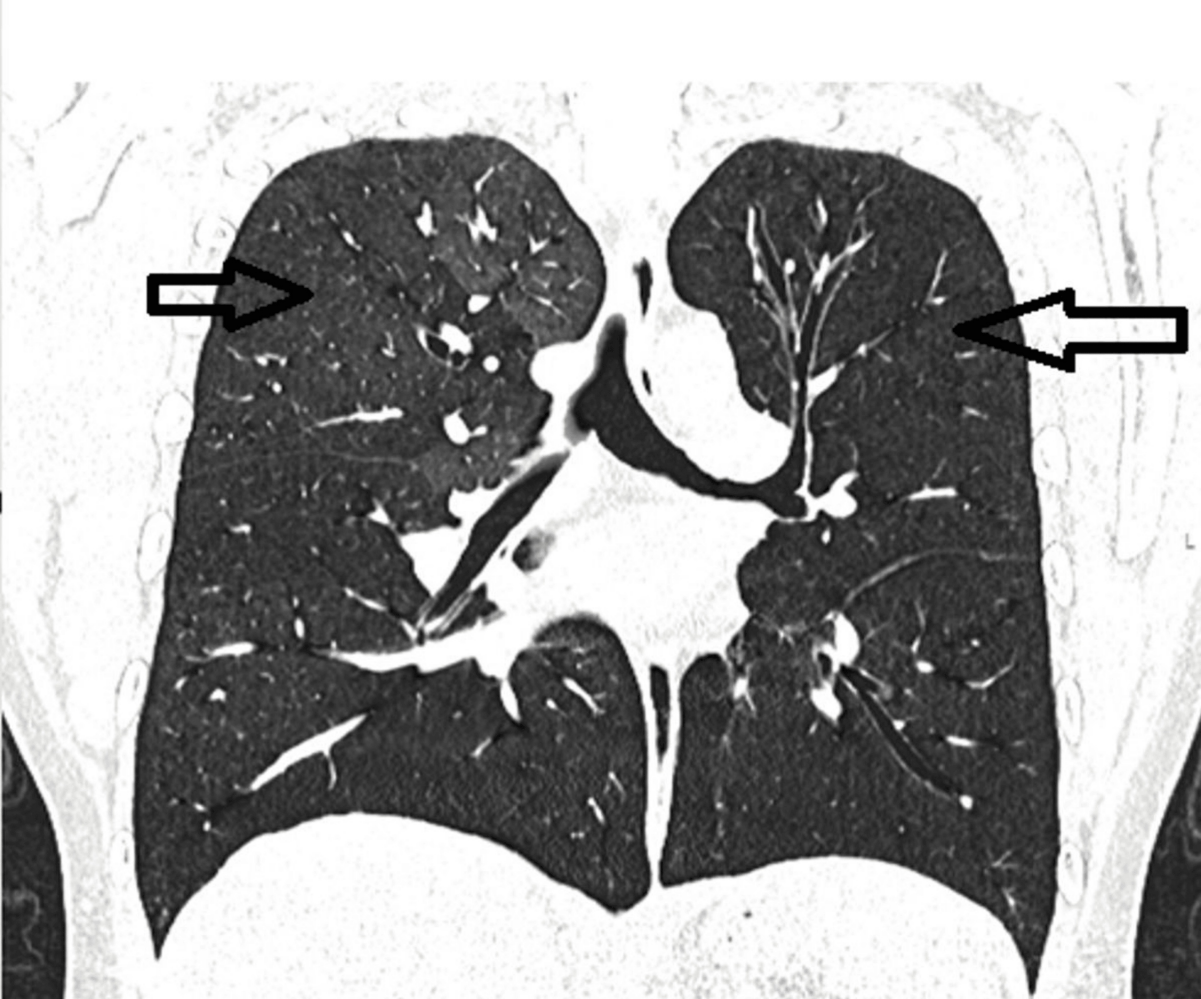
High-resolution computed tomography (HRCT) of the chest, coronal section, inspiratory phase
